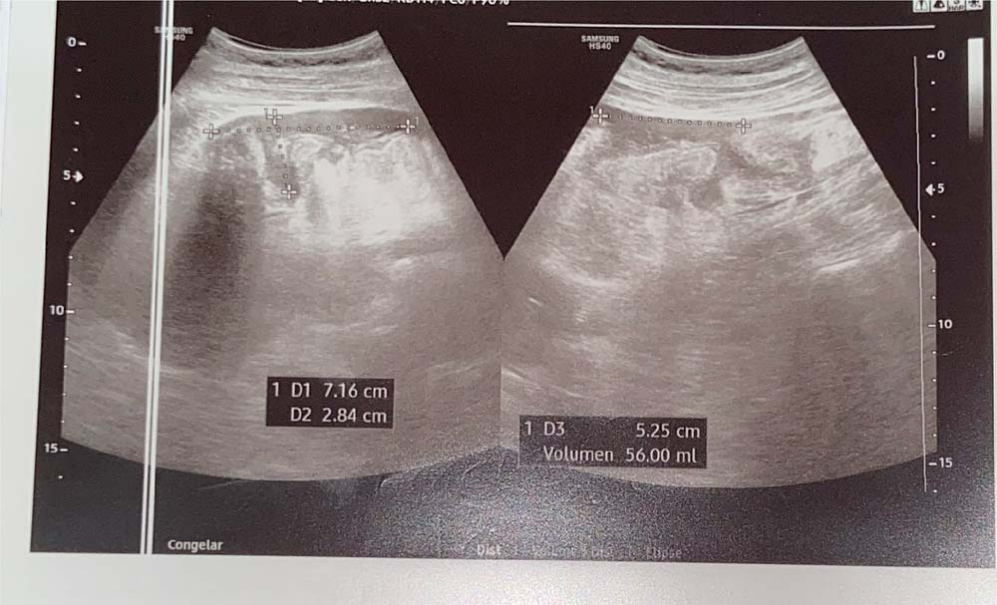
Ultrasound revealing revealed a small amount of free fluid in the perihepatic, perisplenic, and right flank interloop regions.
